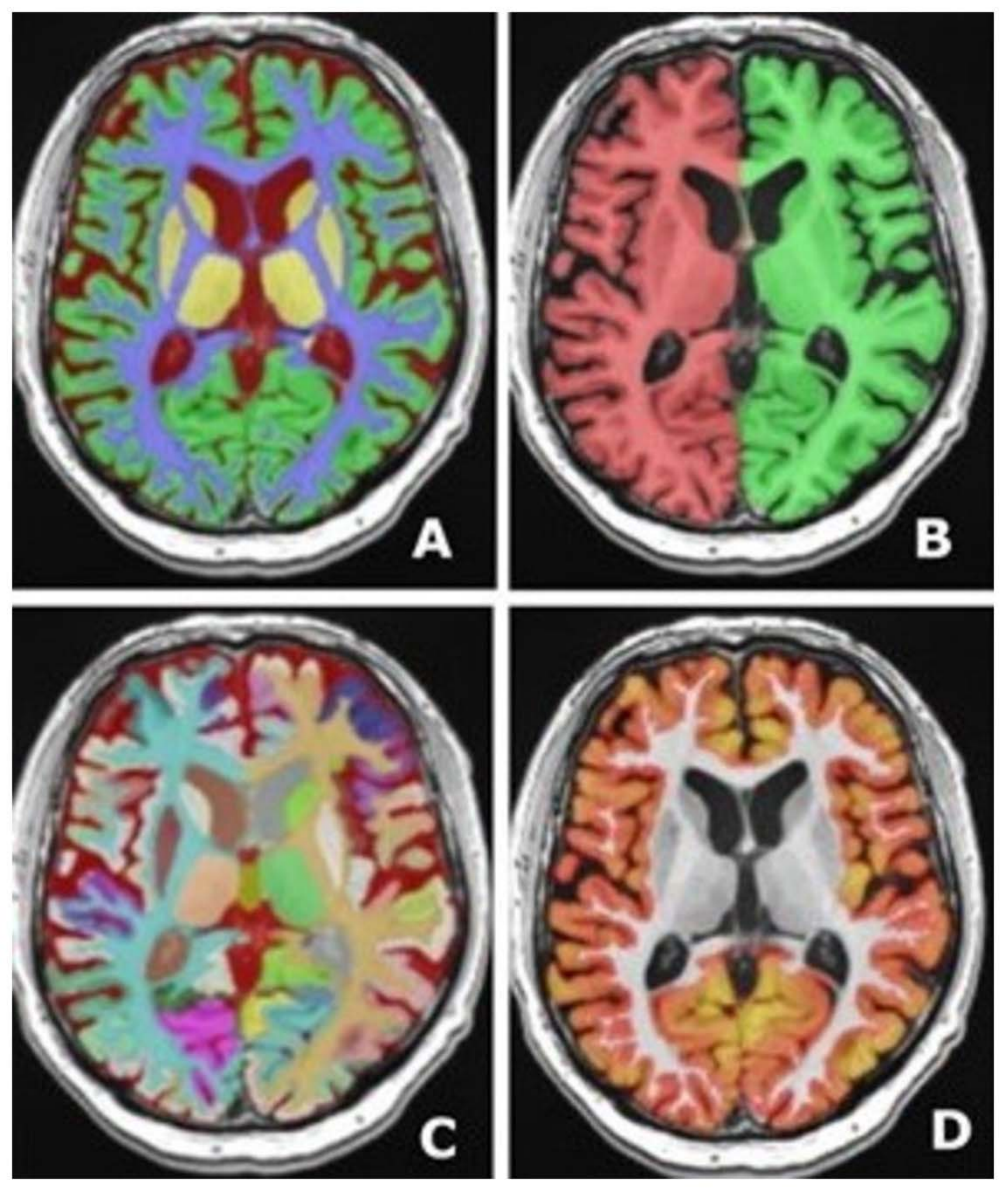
Volumetric analysis using an automated MRI brain volumetry system. (A) Tissue segmentation – for volumetric assessment of white and gray matter; (B) Macrostructures – for volumetric assessment of the hemispheres, and their white and gray matter volume; cerebellar volume is also included; (C) Structure segmentation – detailed volume assessment of each gyrus, and basal ganglia; (D) Cortical thickness – for the evaluation of cortical volume in different regions. MRI: Magnetic resonance imaging.
